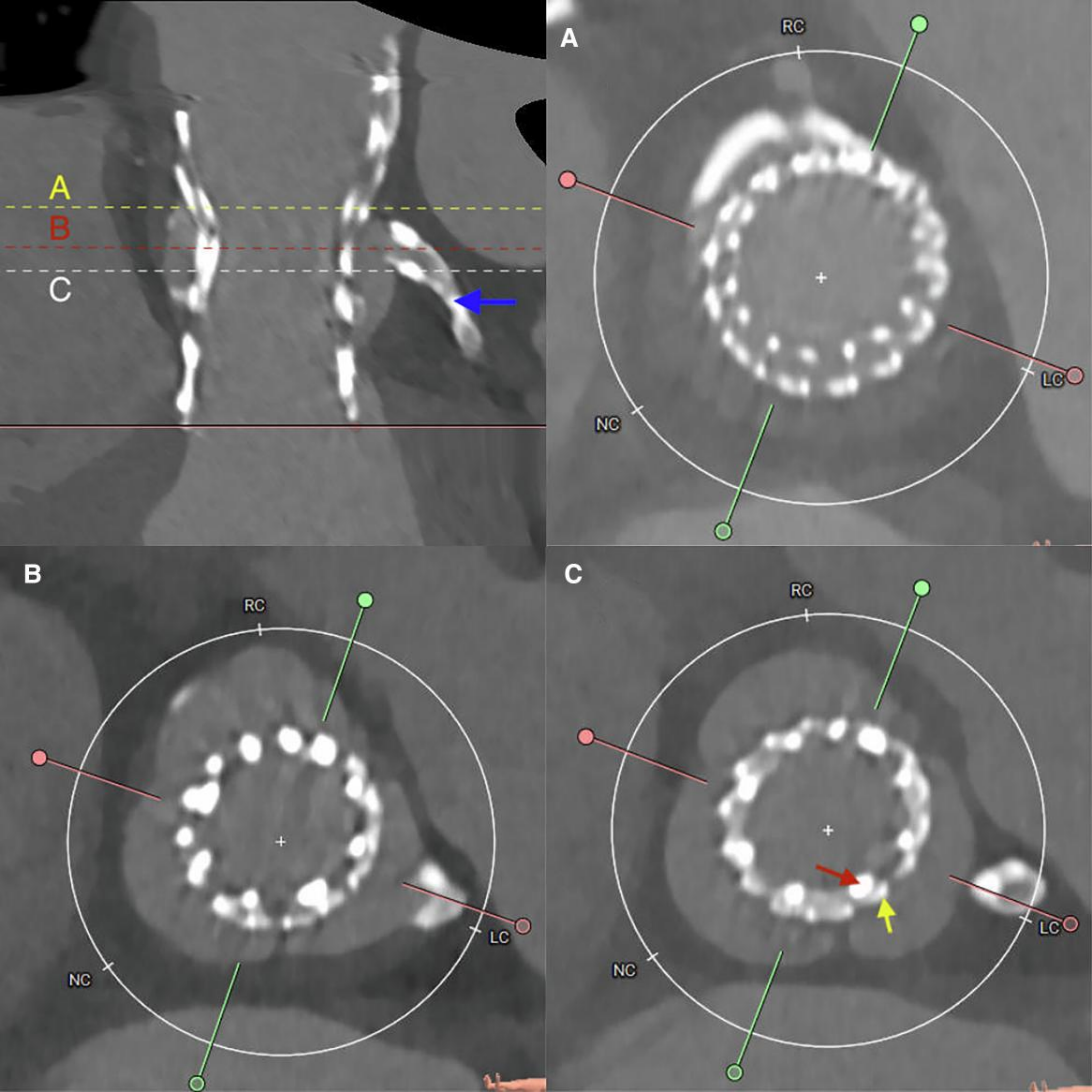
The image depicts aortic and valve dimensions after implantation of the Navitor™ THV. Upper left: depicts a stretched vessel projection of the aortic valve in the plane of the LCA (LCA marked with an arrow). (*A*) Cross-section at the level of the STJ. Note that there is no space in between aorta and the expanded index valve. (*B*) Cross-section at the level of the offspring of the left coronary artery. VTC was initially 7.9 mm for the LCA and 8.7 mm for the RCA, respectively, and remained unchanged after implantation of the Navitor™ THV. (*C*) Cross-section of the level of the highest point of the projected neoskirt, i.e. at 26.2 mm above the lowest point of the index Evolut. Note that the neoskirt and its actual height after implantation of the Navitor™ THV cannot be distinguished on the CCT scan. The red arrow depicts the stent frame of the implanted Navitor valve, the yellow arrow shows the stent frame of the index Evolut™ THV. STJ, sinotubular junction; VTC, valve-to-coronary distance; LCA, left coronary artery; RCA, right coronary artery; THV, transcatheter heart valve; CCT, cardiac computed tomography.
